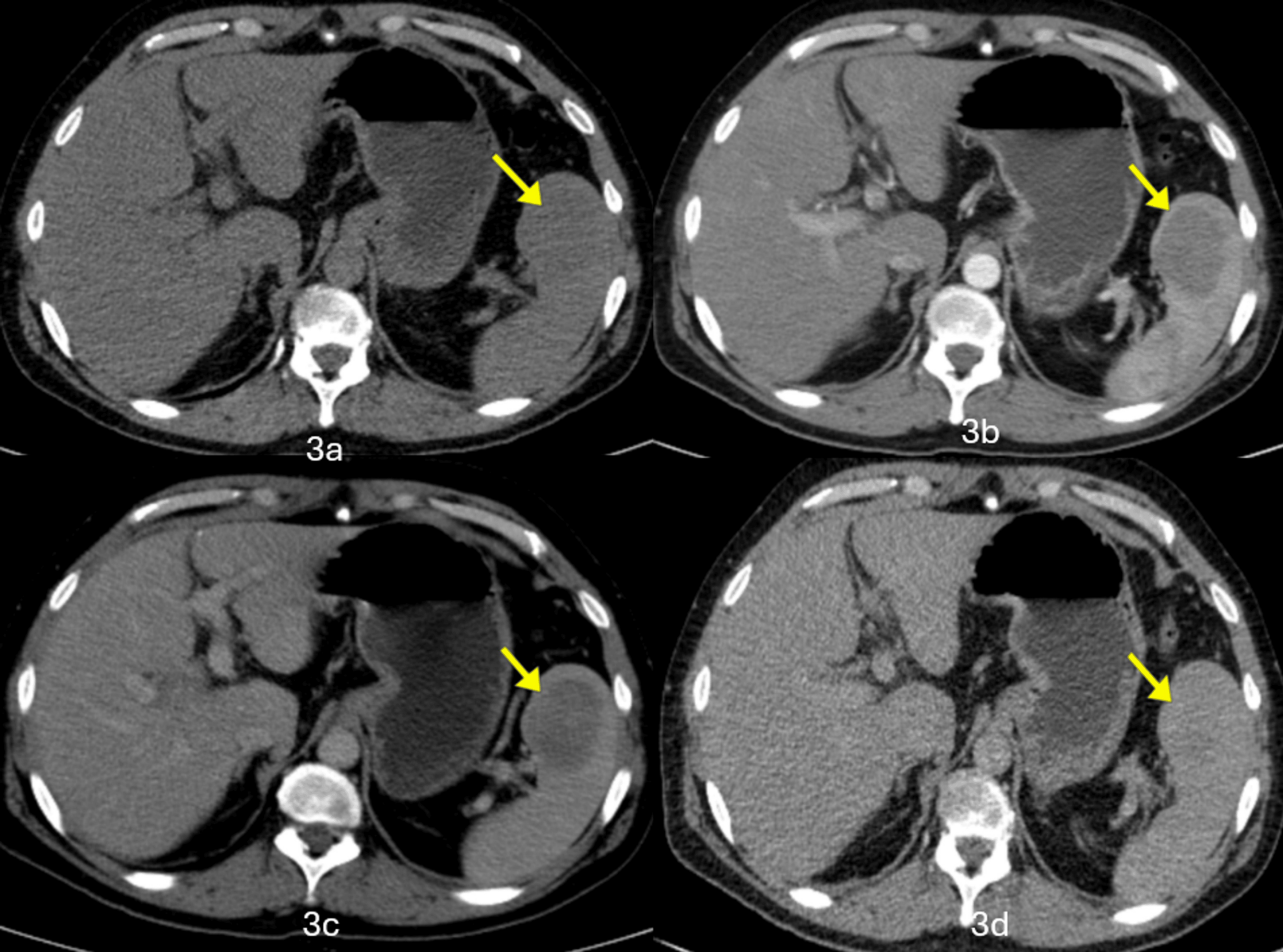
CECT of the abdomen CECT showing a well-defined hypodense lesion in lower pole of the spleen, measuring 4.8 x 3.3 cm (yellow arrow) (3a) with peripheral arterial enhancement (3b) and progressive centripetal filling in the venous (3c) and delayed (3d) images, producing a characteristic "spoke-wheel" pattern of enhancement. CECT, contrast-enhanced computed tomography
